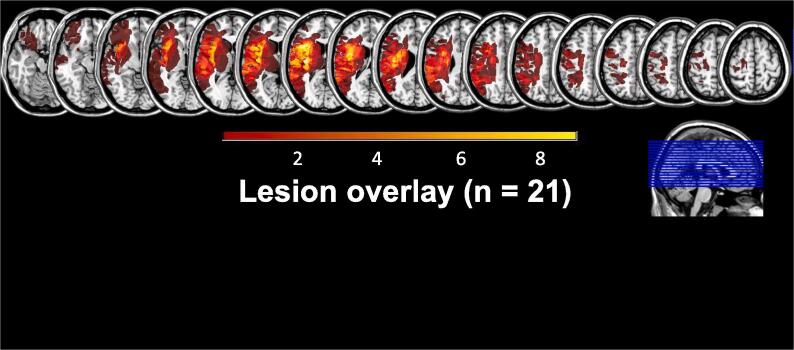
Lesion overlay. The lesion overlay for 21 of the 24 LH stroke patients is shown. One patient did not give informed consent to obtain the clinical CT/MRI images for lesion mapping, and for two patients, no clear lesion demarcation in early clinical imaging was detectable for lesion mapping despite persistent deficits. The overlay indicates that all patients suffered a single LH stroke affecting the left middle cerebral artery (MCA) territory. Color shades represent the increasing number of overlapping lesions. Slices are shown with MNI- coordinates ranging from −17 to 63.
